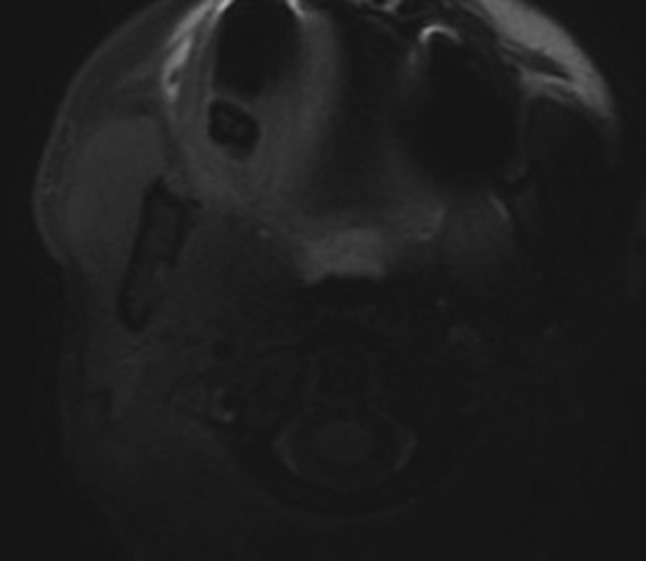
Cervical MRI, axial fat suppressed SE T1-weighted sequence. Studying the cervical portion of left carotid artery is hampered by magnetic susceptibility artifact due to the presence of dental implant.
